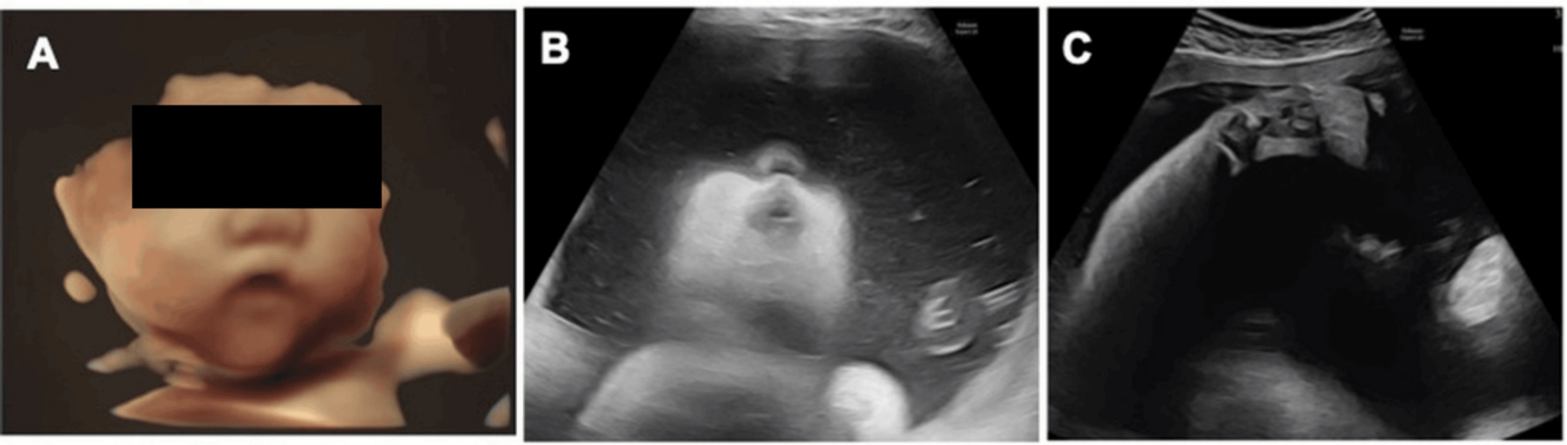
Fetal ultrasonographic findings at 33 weeks of gestation (A, B) Micrognathia; (C) Clubfoot
